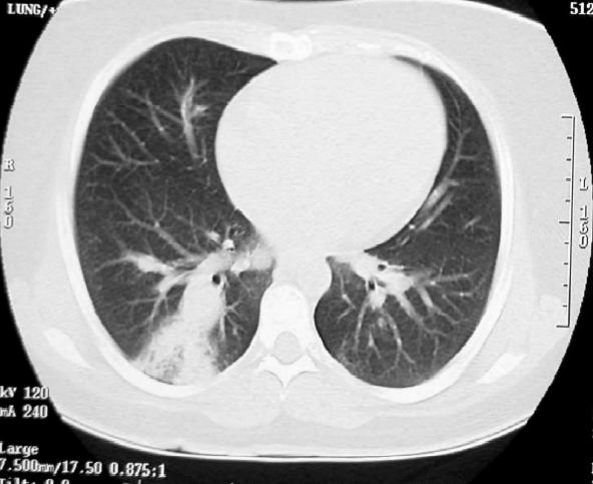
CT scan of the chest showing ill defined infiltrate in lower lobe of the right lung. Reproduced with permission from Al-Jahdali H [15].
